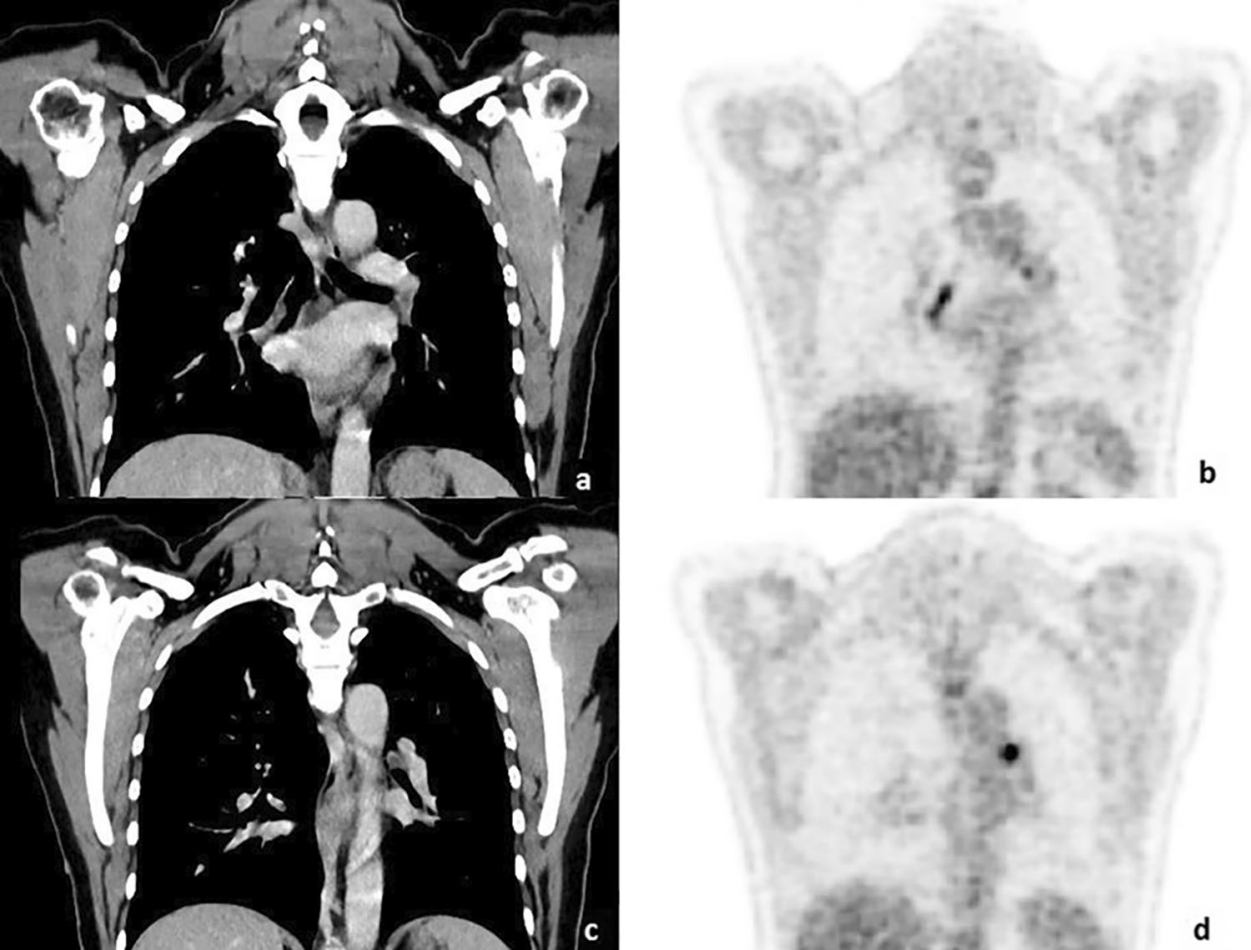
Suspected hilar lymph node metastases; not confirmed in imaging follow-up. a CT imaging of LN in a coronary slice b PET imaging corresponding to (a). c CT imaging of LN in a different coronary slice of the same patient. d PET imaging corresponding to (c)
